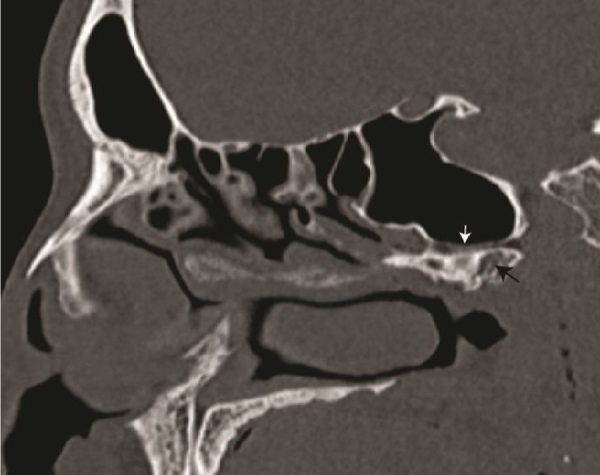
Sagittal computed tomography scan slide showing the vidian canal (white arrow) and a pneumatised pterygoid process (black arrow).
